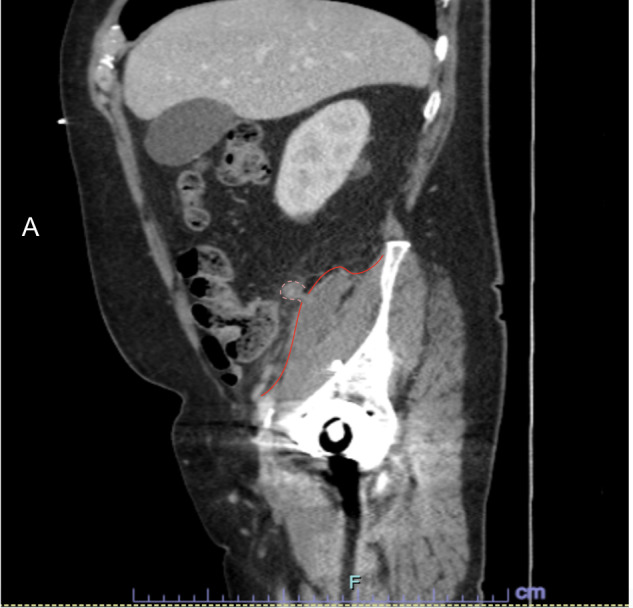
Sagittal view CT depicting the appendix fistulized to the iliopsoas accompanied by abscess formation. White dotted line: border of the appendix. Red line: border of the iliopsoas.
